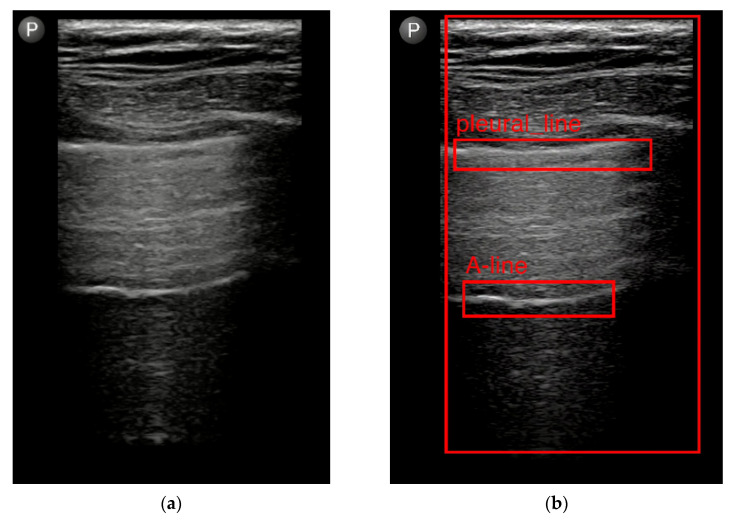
Evaluation of the lung ultrasound video using artificial intelligence: (a) Lung ultrasound video from linear probe with A-lines; (b) Visualization of anatomical landmarks (pleural line) and ultrasound sign (A-line) after evaluation of the same video as in (a) by artificial intelligence using analytical approach [49].
